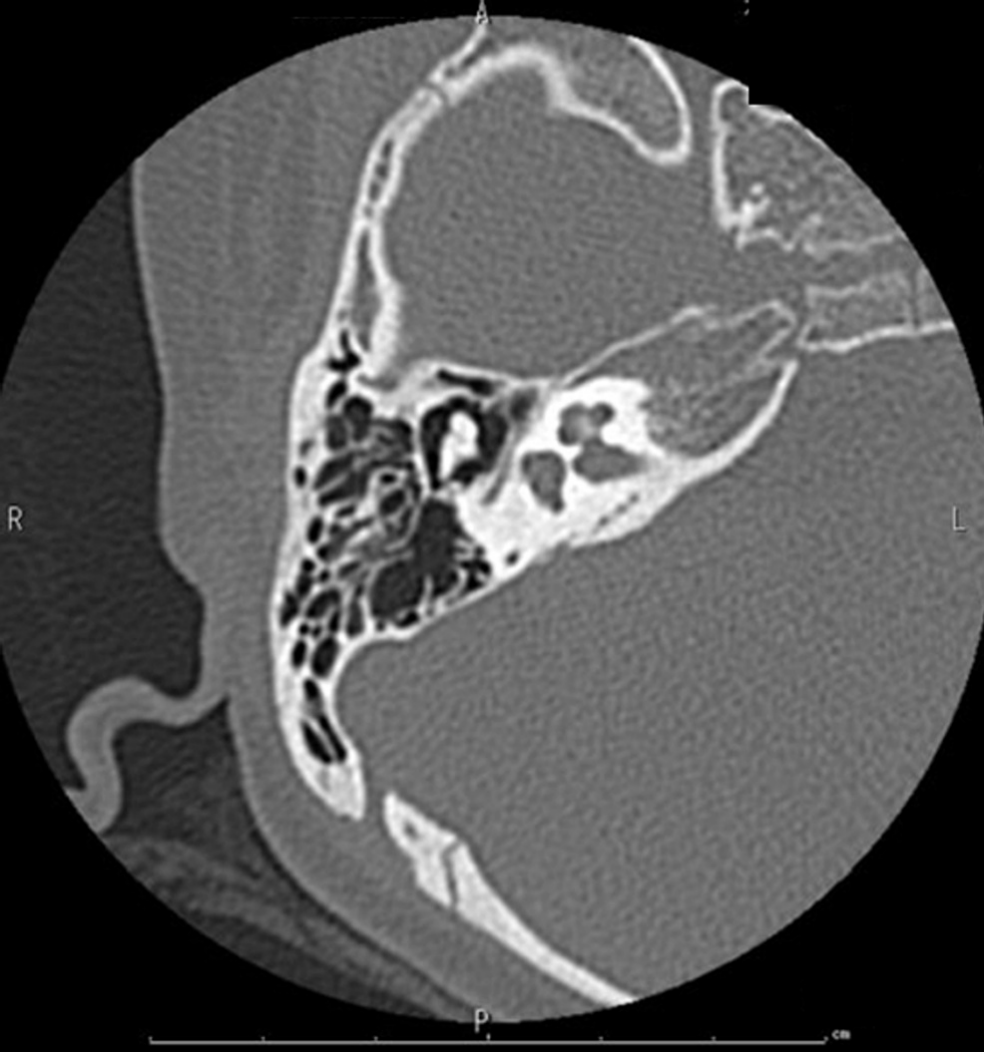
Imaging of Case 1: temporal bone CT (right) Affected side. CT, computed tomography.
